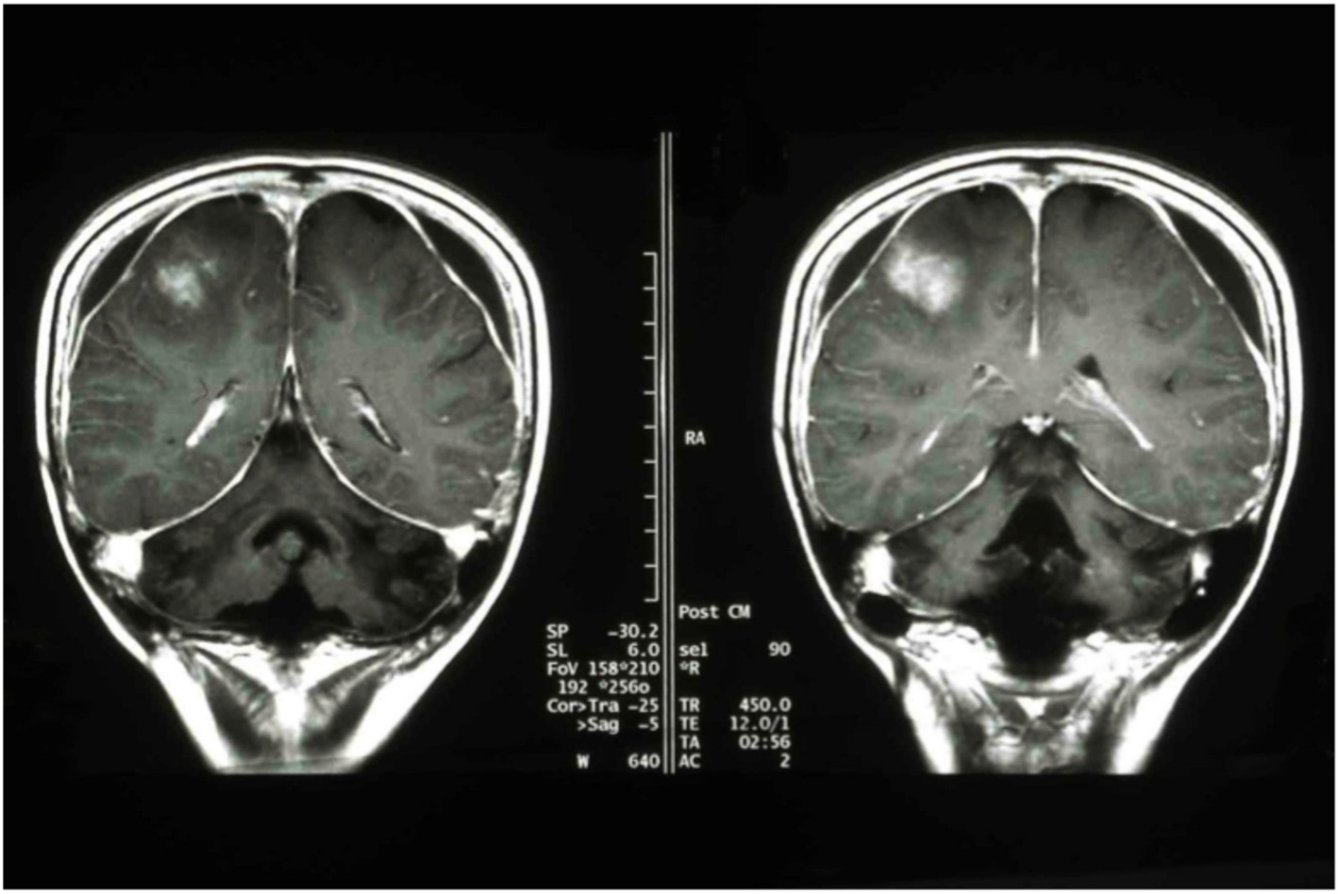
Brain MRI shows new enhanced lesion in the right frontoparietal lesion.
